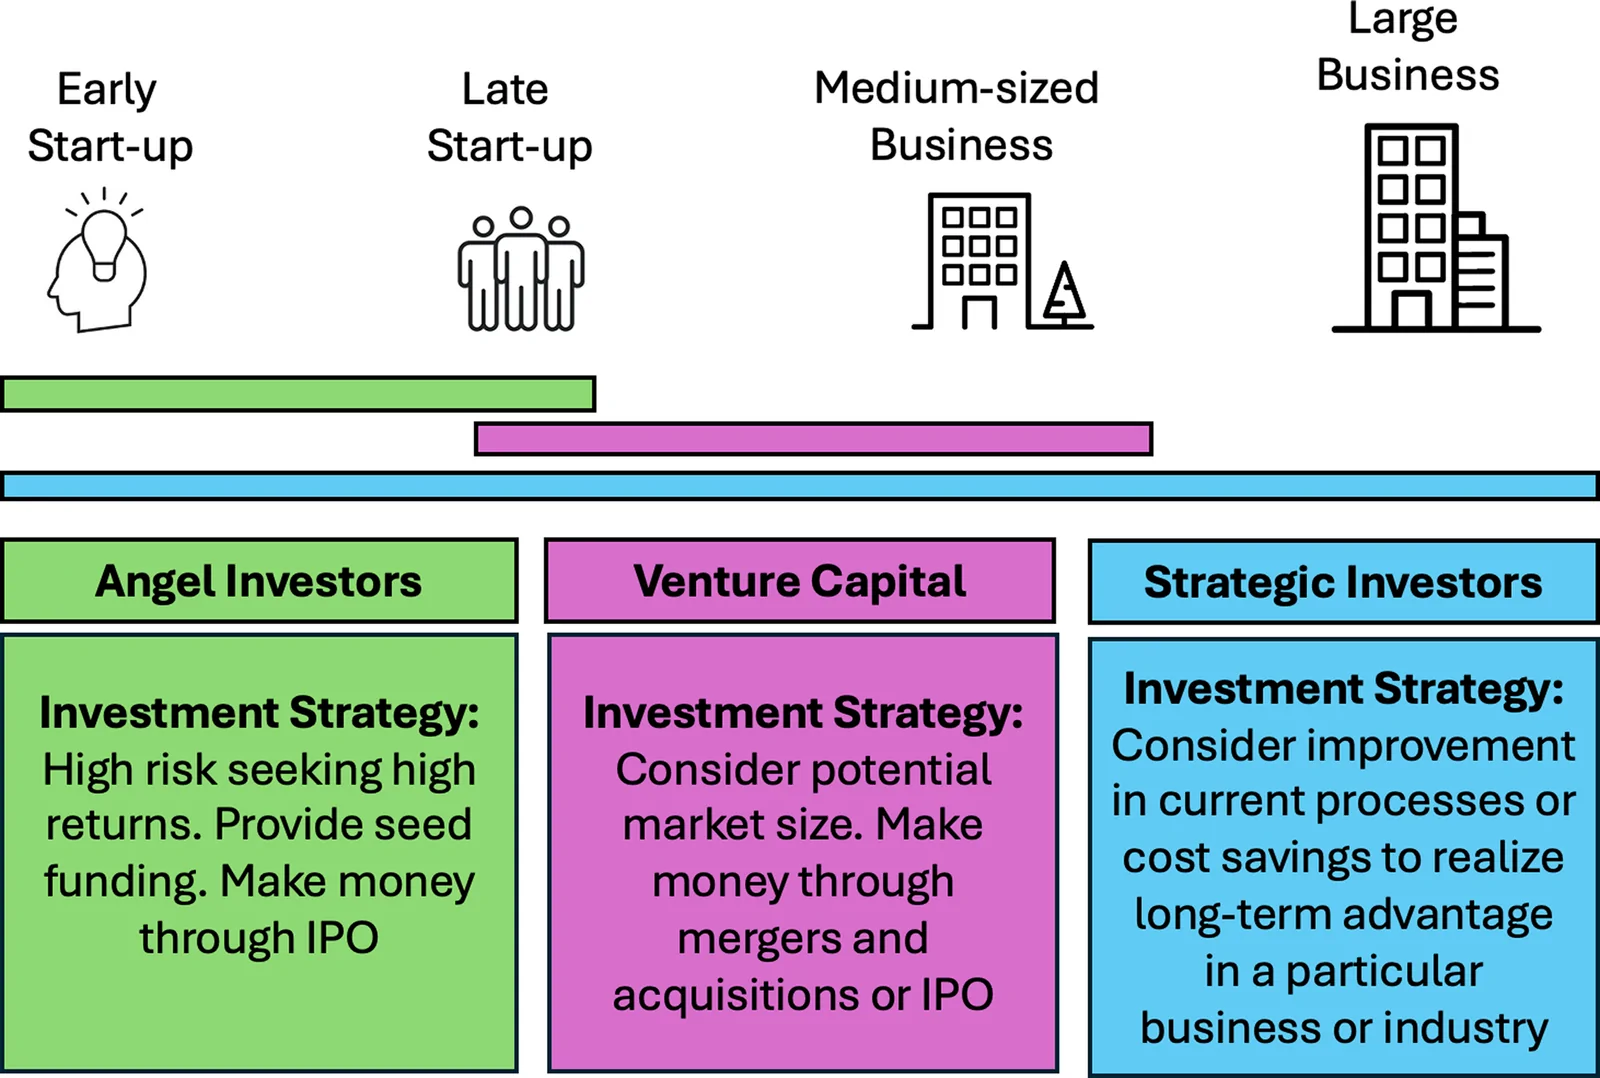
Simplified investor and investment strategy for point-of-care ultrasound artificial intelligence product development. IPO, initial public offering.
